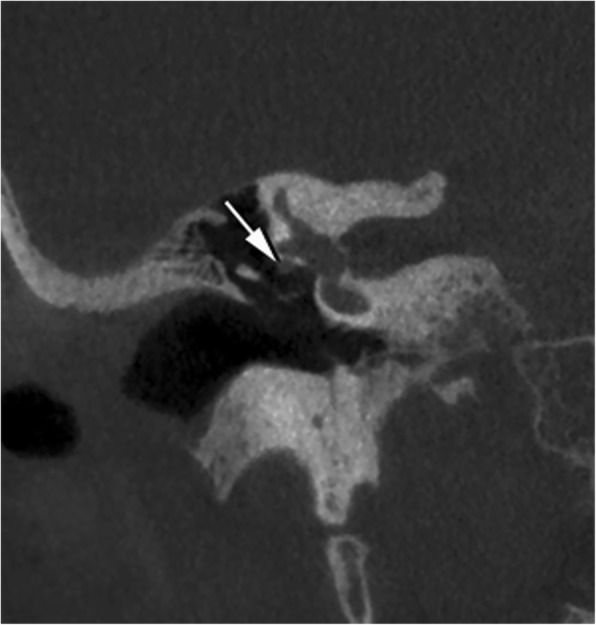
Facial nerve canal dehiscence. Coronal CBCT reconstruction shows dehiscence of the canal and minimal protrusion of the facial nerve (arrow) within the middle ear cavity
